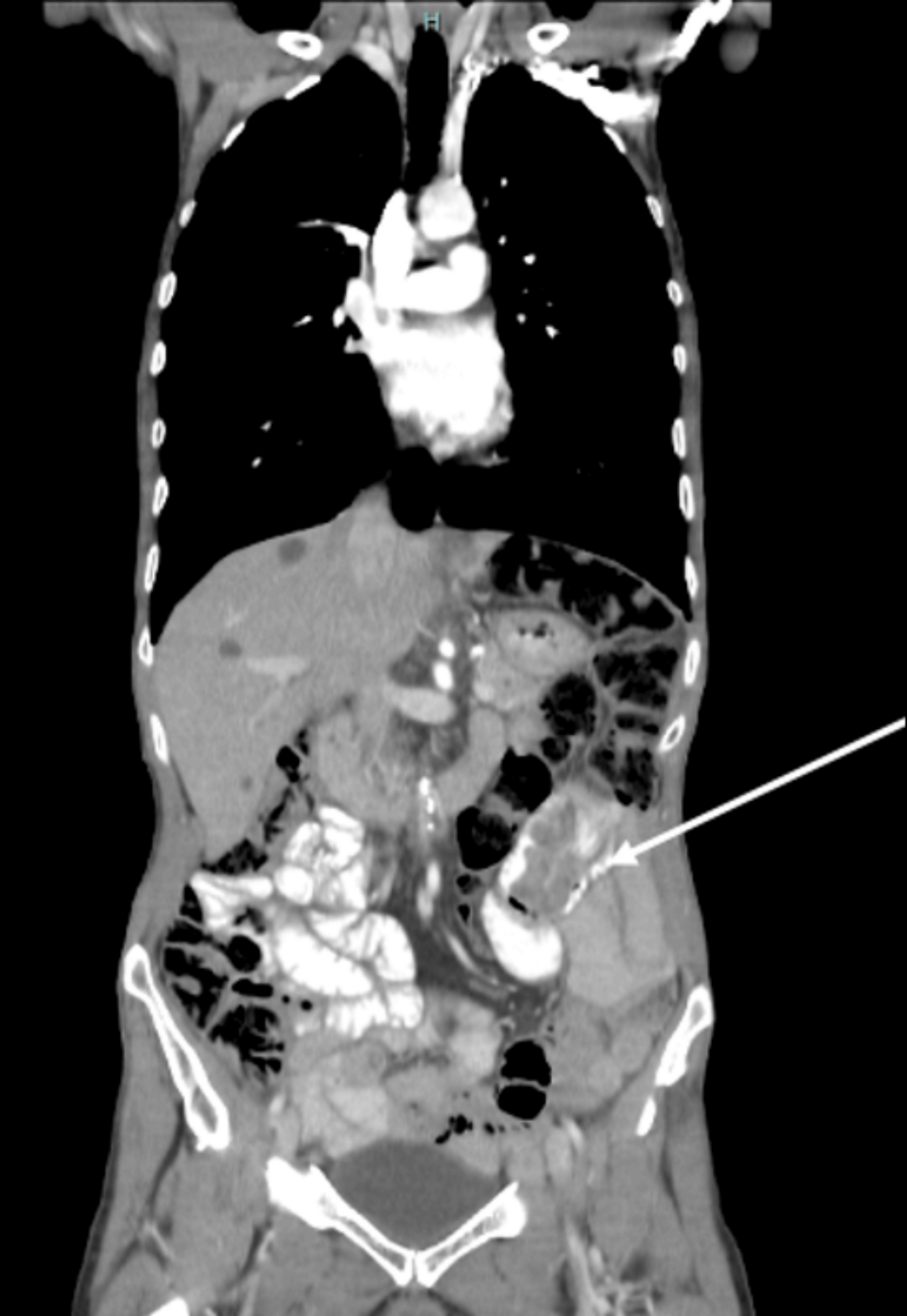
Abdominal CT demonstrating an endoluminal jejunal lesion (coronal view)
